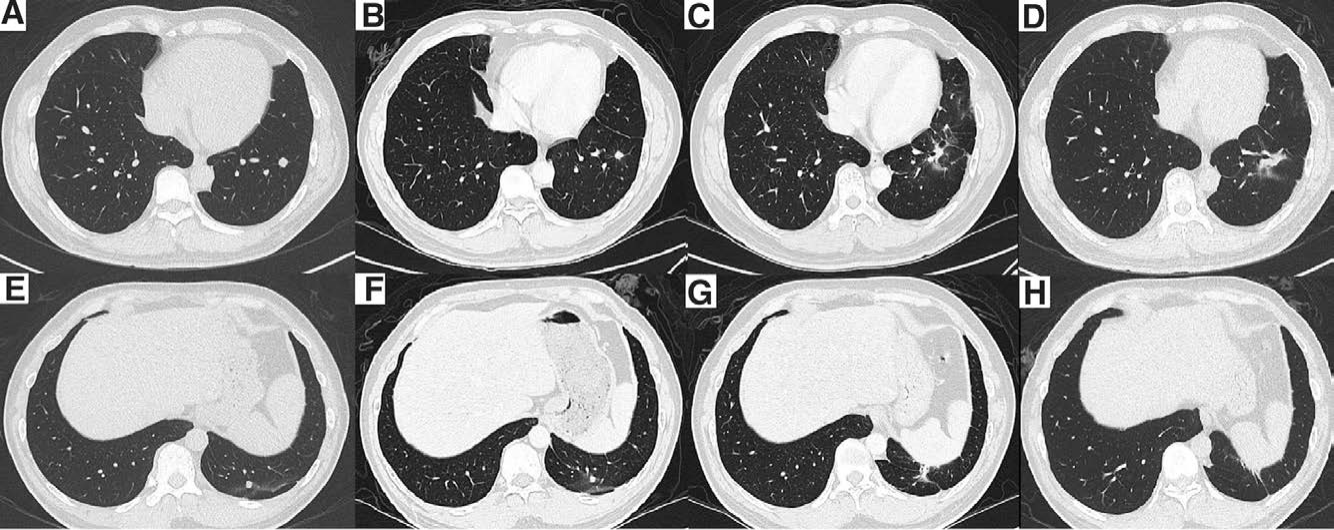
Serial images of chest CT. CT scans of upper lesion at pre-SABR (A), one month after SABR (B), seven months after SABR (C) and fourteen months after SABR (D). CT scans of lower lesion at pre-SABR (E), one month after SABR (F), seven months after SABR (G) and fourteen months after SABR (H). CT = computed tomography, SABR = stereotactic ablative radiotherapy.
